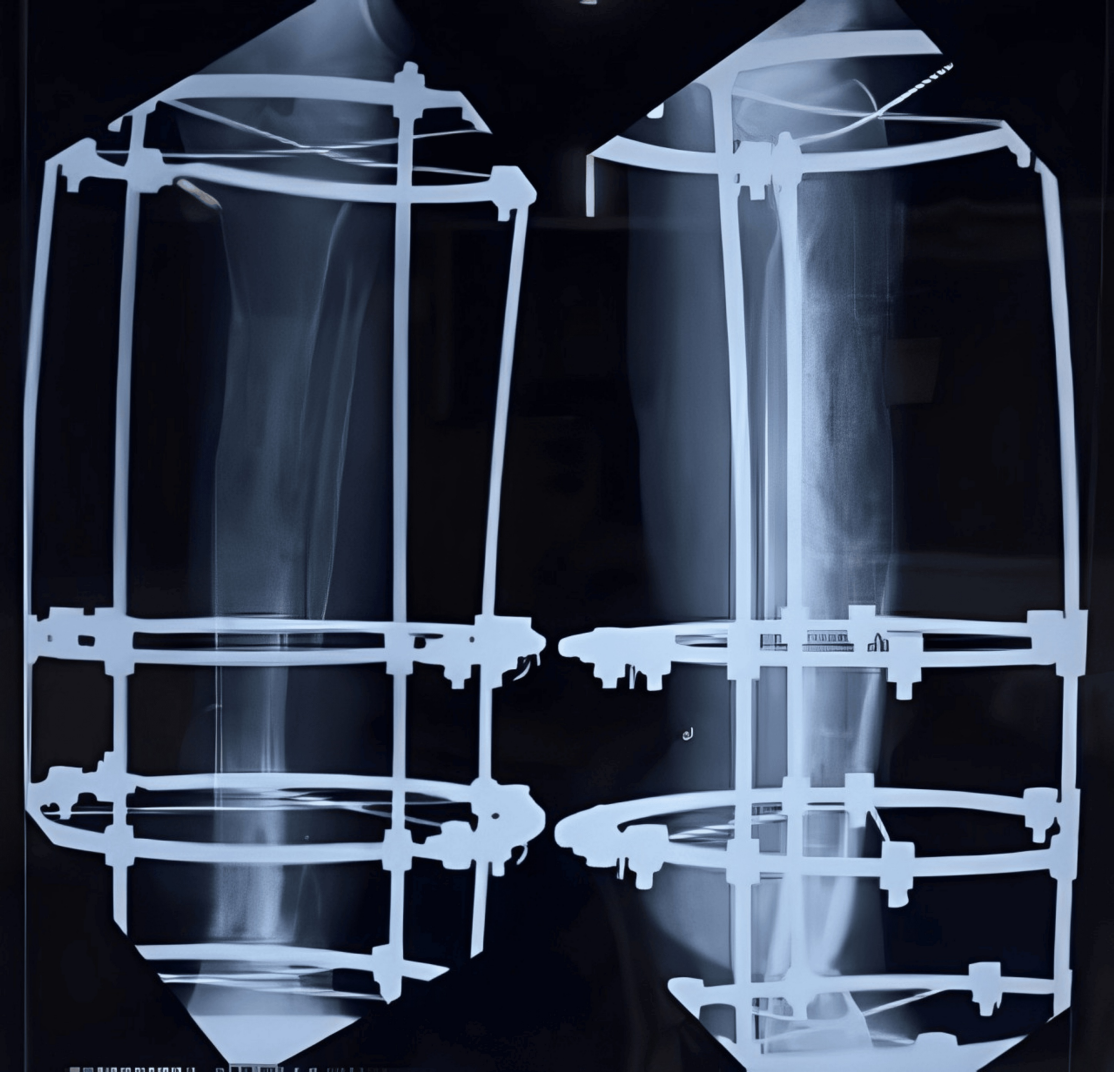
X-ray after six months of stopping the distraction showing a well-healing consolidation
